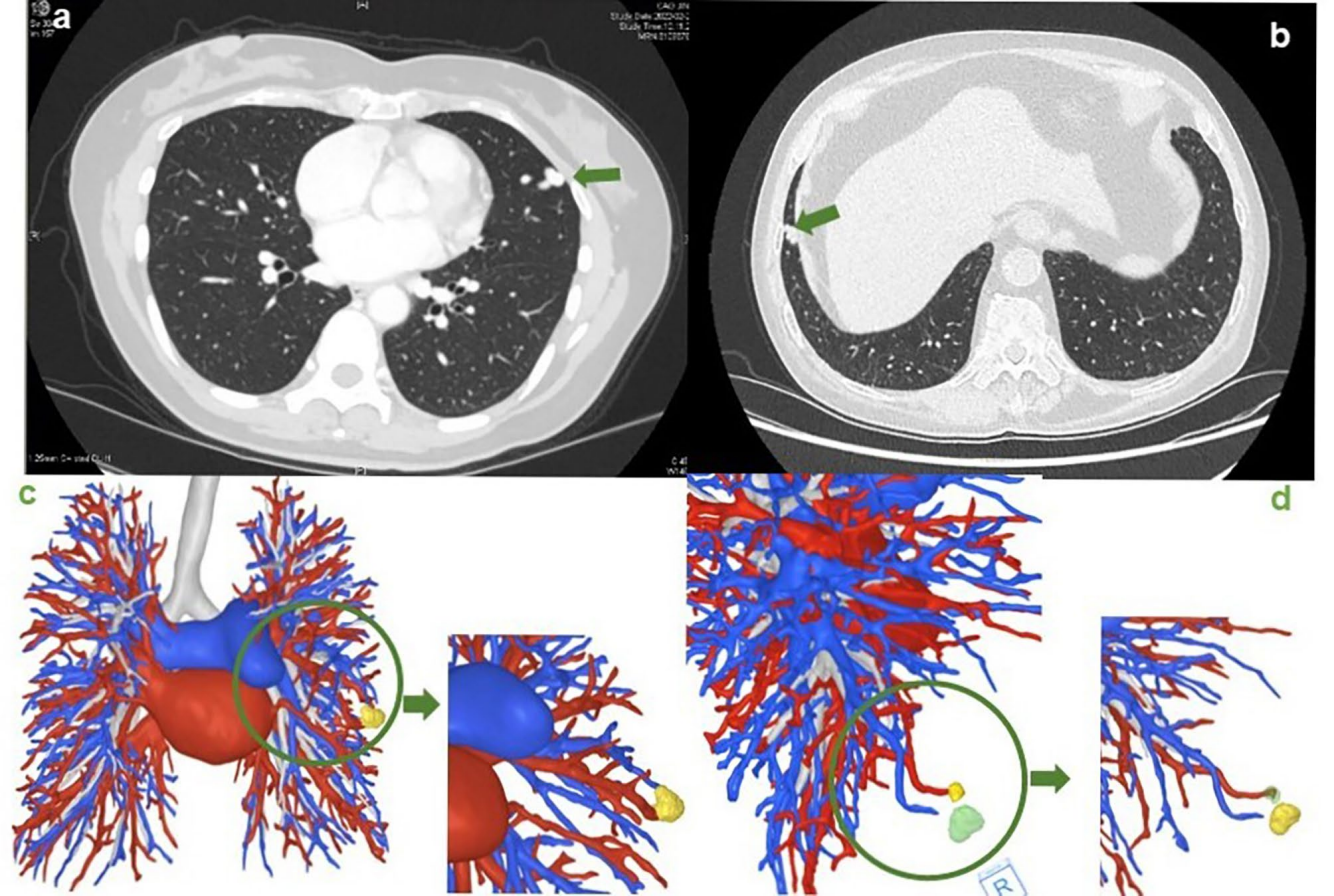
CT and 3-dimensional imaging for two cases. a. CT view of the first patient shows a nodular opacity in the lingual segment of the left upper lobe; b. CT view of the second patient shows a nodule in the anterior basal segment of the right lower lobe c. 3-dimensional imaging of the first patient’s lung vasculature; d. 3-dimensional imaging of the second patient’s lung vasculature
